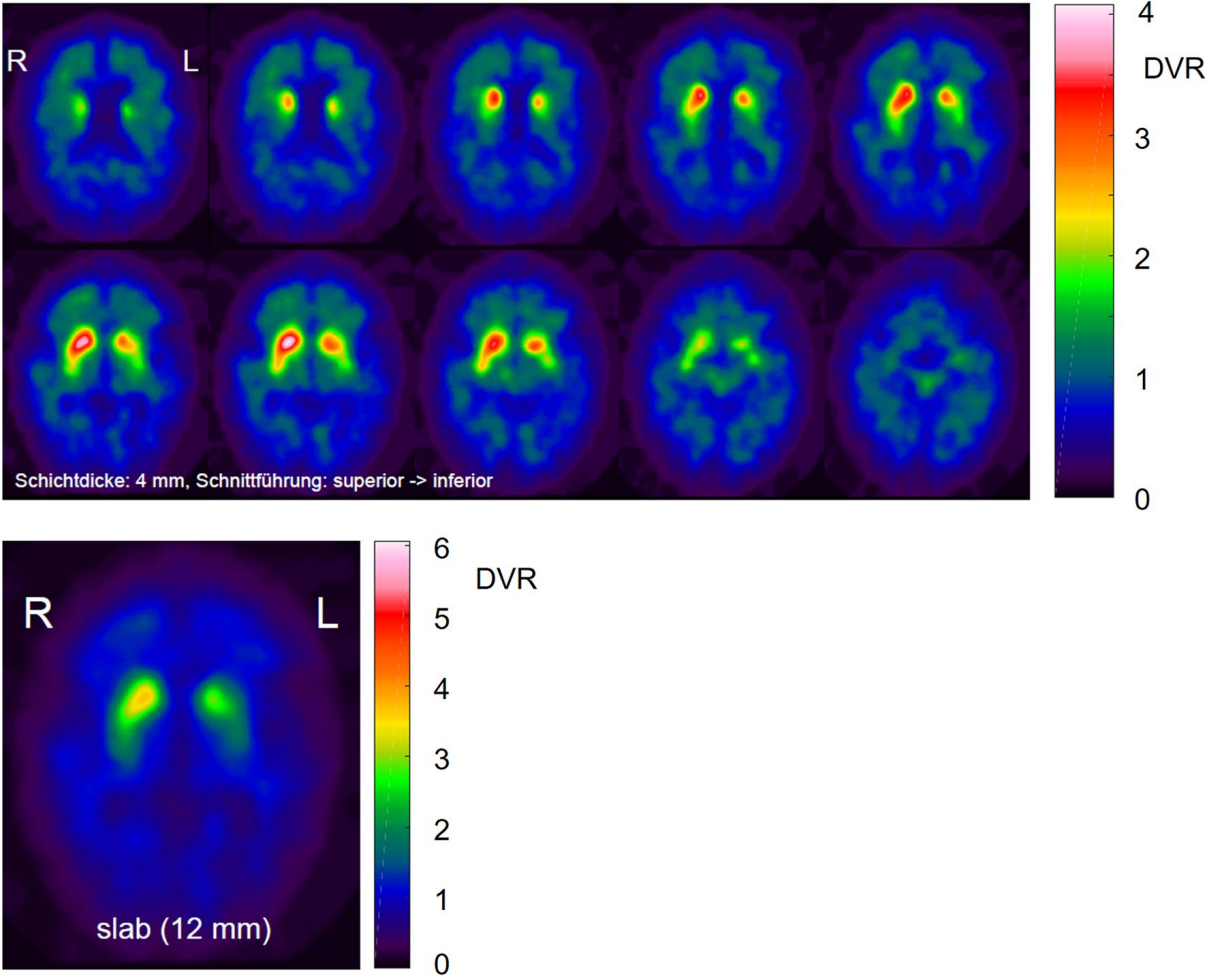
Standard display for visual interpretation of the DAT-SPECT images. Standard display for visual interpretation of the DAT-SPECT images presenting ten transversal distribution volume ratio (DVR) image slices of 4 mm thickness from the superior to the inferior edge of the striatum with the maximum of the colortable individually scaled to the maximum intensity in the ten images. In addition, the display presents a transversal DVR image slab of 12 mm thickness through the center of the striatum with the maximum of the colortable scaled to a fixed DVR threshold [25]. The DVR threshold had been optimized previously, separately for each of the three settings (MPH, LEHRH-FBP, LEHRHS-OSEM)
